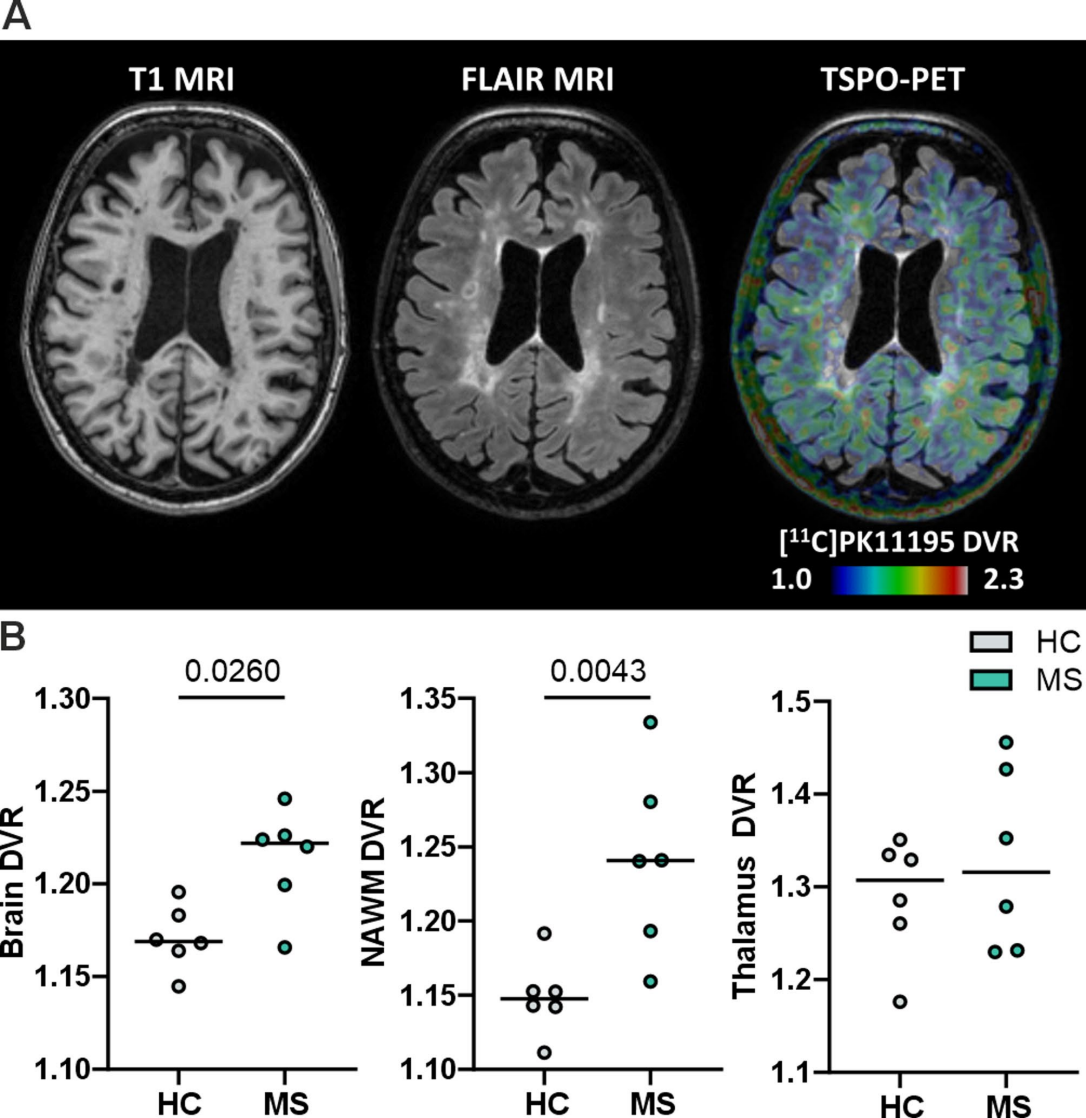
^11^C-PK11195-PET brain imaging of pwMS and HCs. A T1-weighted, FLAIR and TSPO-PET images of a patient with MS show a significant lesion load and increased innate immune cell activation, as evidenced by increased [^11^C]PK11195 binding in the NAWM and perilesional areas. The colour bar shows the dynamic range of the DVR in the PET image. B PET-measured brain DVR, NAWM DVR and thalamus DVR of the HCs (*n* = 6) and pwMS (*n* = 6) included in this study. The data are presented as single datapoints and medians. Significance was determined with the Mann–Whitney U test
